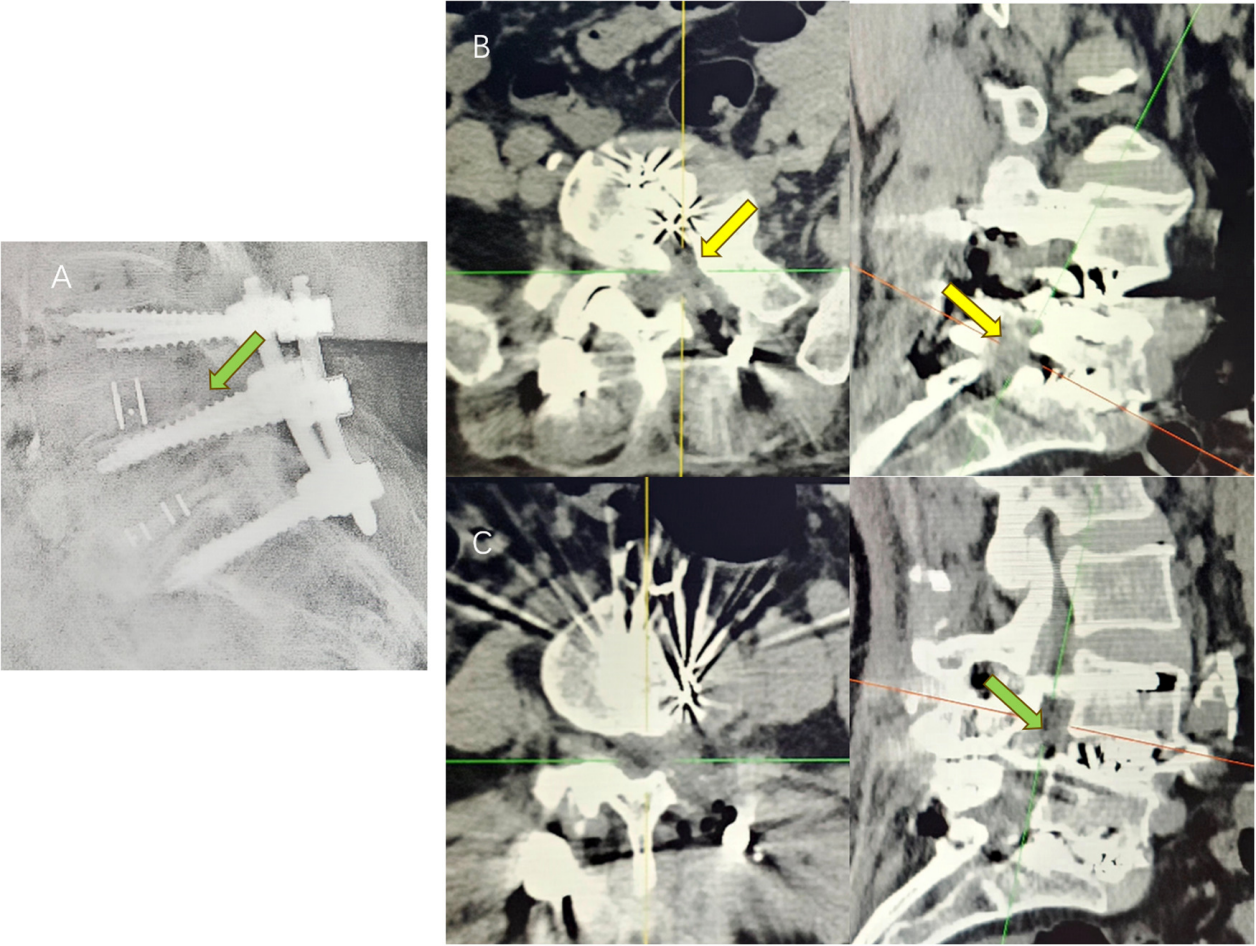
(A) postoperative DR showed that the intervertebral height of the L5/S1 segment was restored and the L4 slip was corrected; (B) postoperative CT showed that the cyst on the left side of the L5/S1 had disappeared (yellow arrow), and the intervertebral height of the corresponding segment was restored; (C) postoperative CT showed that the L4 slip was corrected (green arrow).
